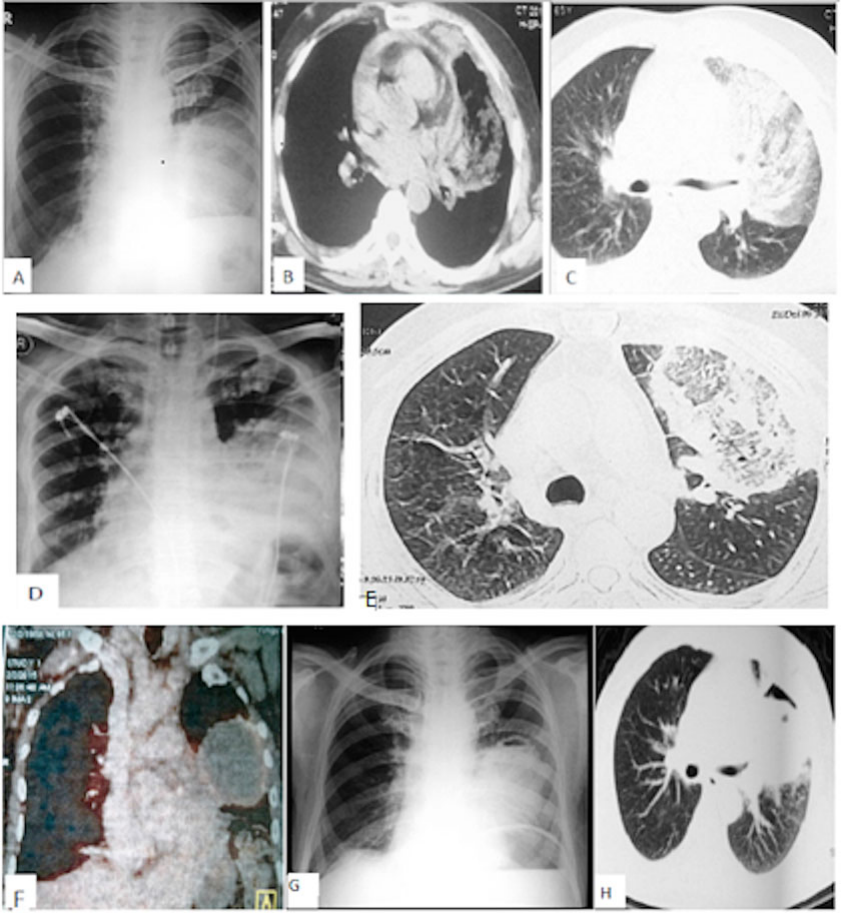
CXR‐PA and CT scan of the thorax showing left upper lobe consolidation (A–C), left upper lobe consolidation with reverse halo sign (D,E), PET CT scan (F) and air crescent sign (G,H).
